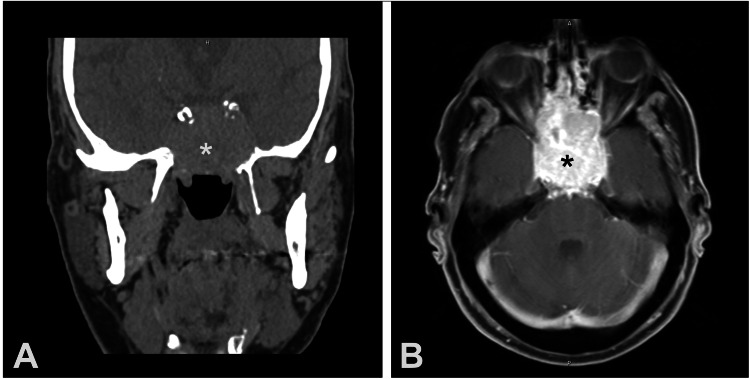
Image depicting RCC metastasis to the sphenoid, posterior ethmoids, sella, and clivus (*) as seen on coronal CT of head (A), image of RCC metastasis to the sphenoid, posterior ethmoids, sella, and clivus (*) as seen on axial MRI of head (B). RCC: Renal cell carcinoma.
